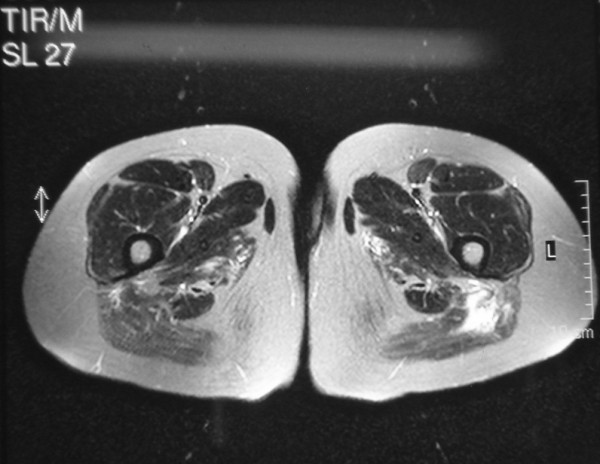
Magnetic resonance imaging (MRI) of the gluteal and posterior femoral muscles revealed increased signal intensity on T1 sequencing, attributed to edema and inflammation. Along with MRI, electromyography and muscle biopsy findings were compatible with the diagnosis of polymyositis
